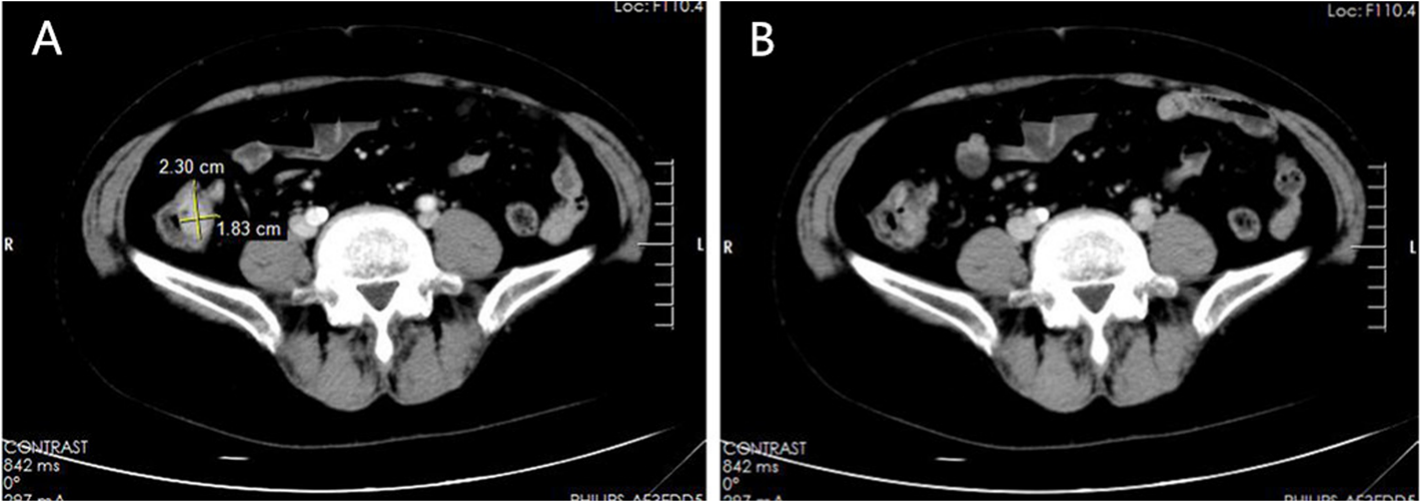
CT scan showed a soft tissue approximately 2.3 cm × 1.8 cm in the ileocecal area with irregular borders (b), and moderately continuous enhancement after enhancement (a)
